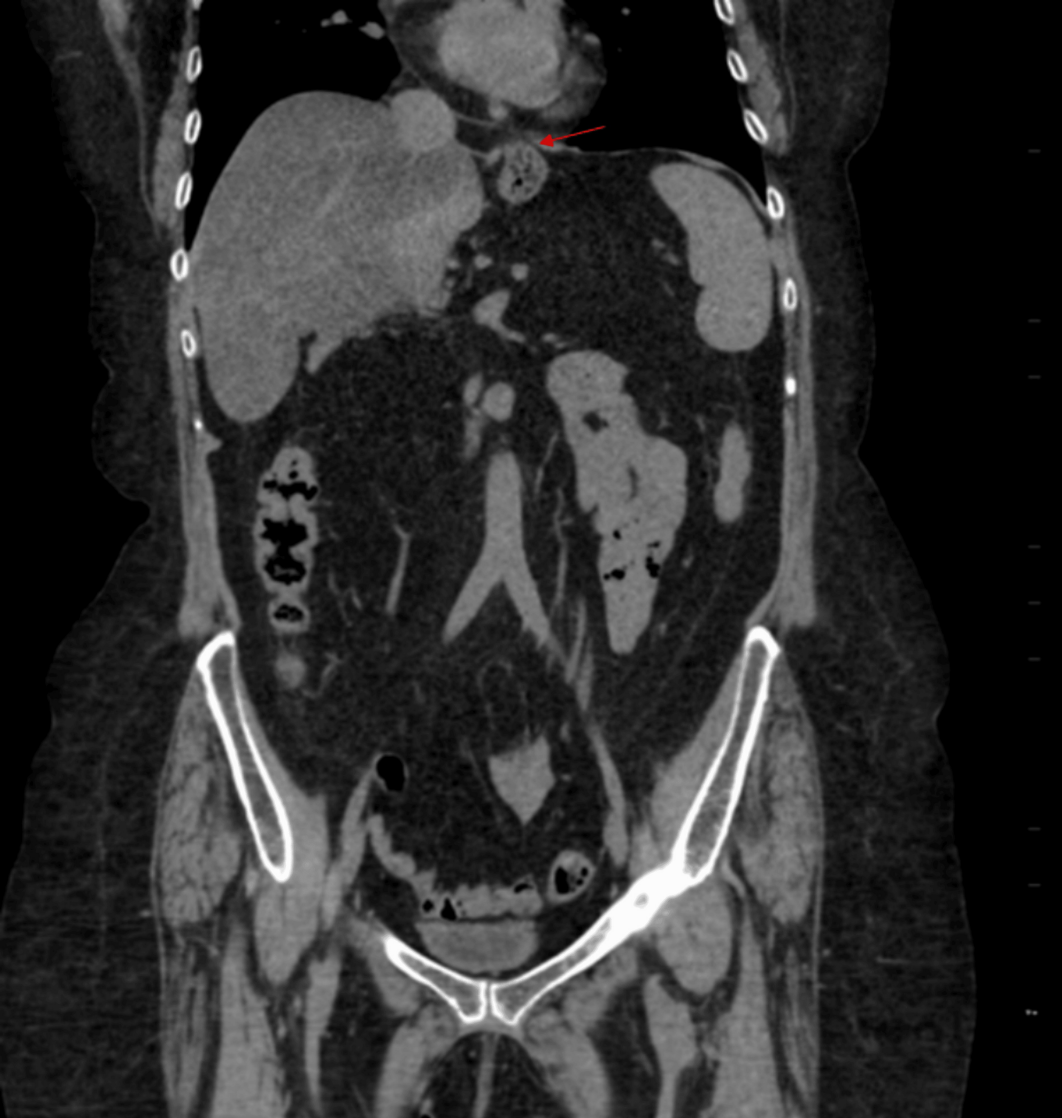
CT abdomen Moderate-sized hiatal hernia (indicated by the red arrow). No acute bowel obstruction. No pneumoperitoneum. No CT evidence of acute colonic diverticulitis. CT: computed tomography
